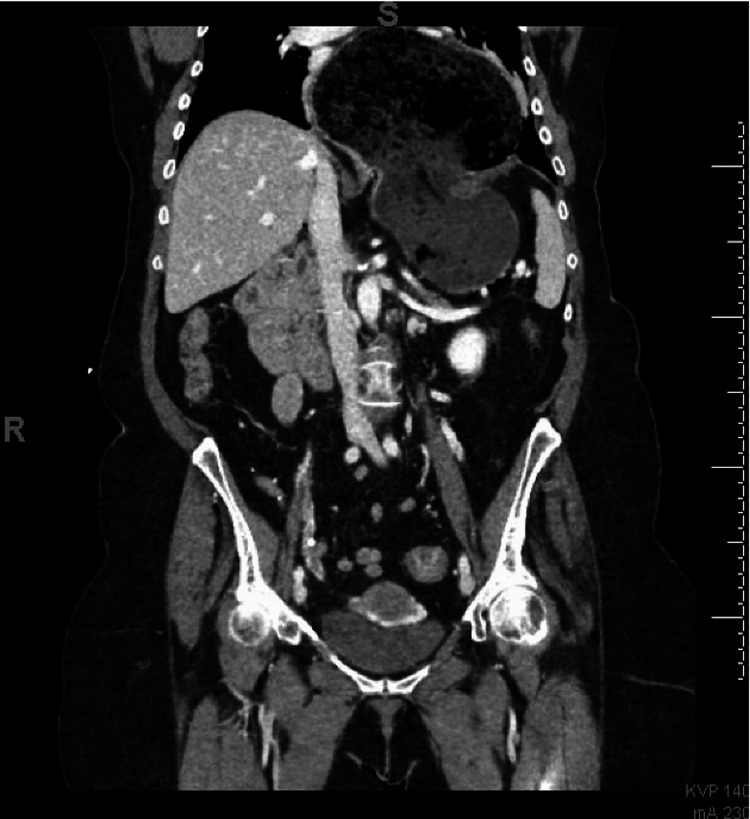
A computed tomography scan of the abdomen and pelvis with contrast revealed hiatal hernia with gastric volvulus and obstruction at the level of the diaphragm.
